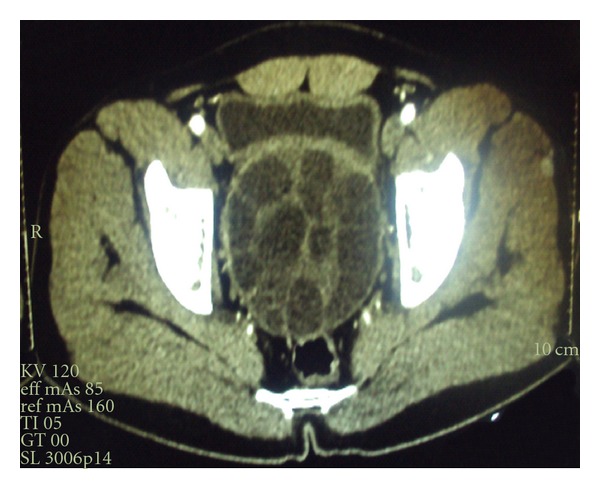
CT showing a pelvic multiloculated hydatid cyst.
